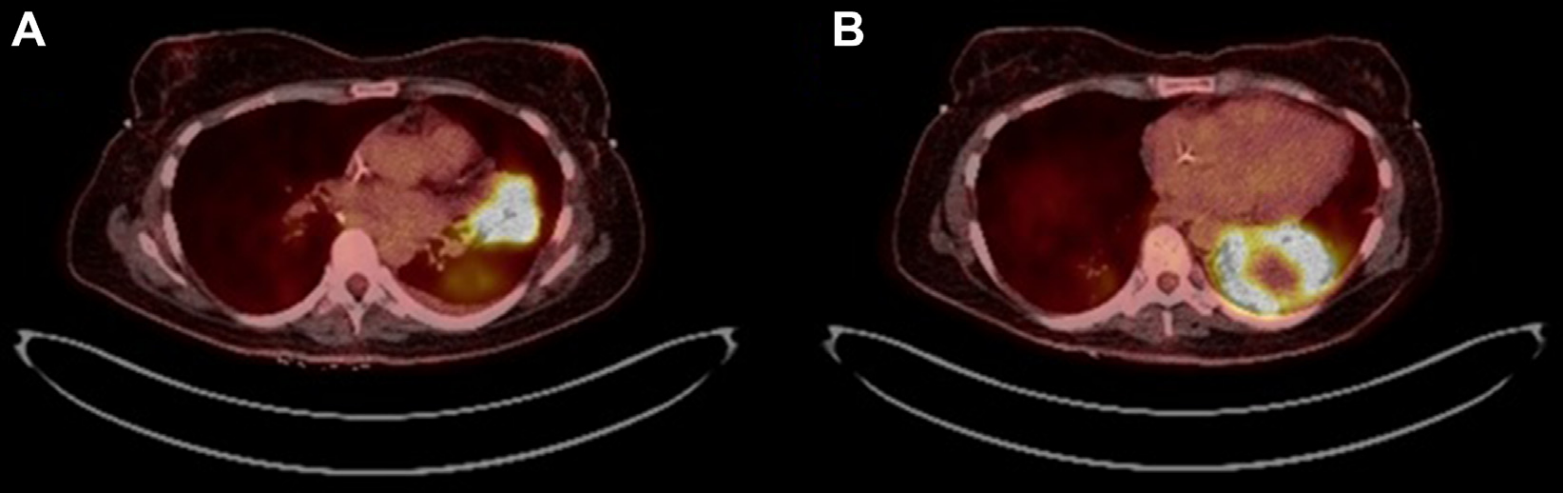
Axial PET images demonstrating left upper lobe mass measuring 4.8 × 3.9 cm with max SUV 10.6 (A) and left lower lobe mass measuring 8.7 × 5.2 cm with max SUV 13.4 (B). SUV = standardized uptake value.
